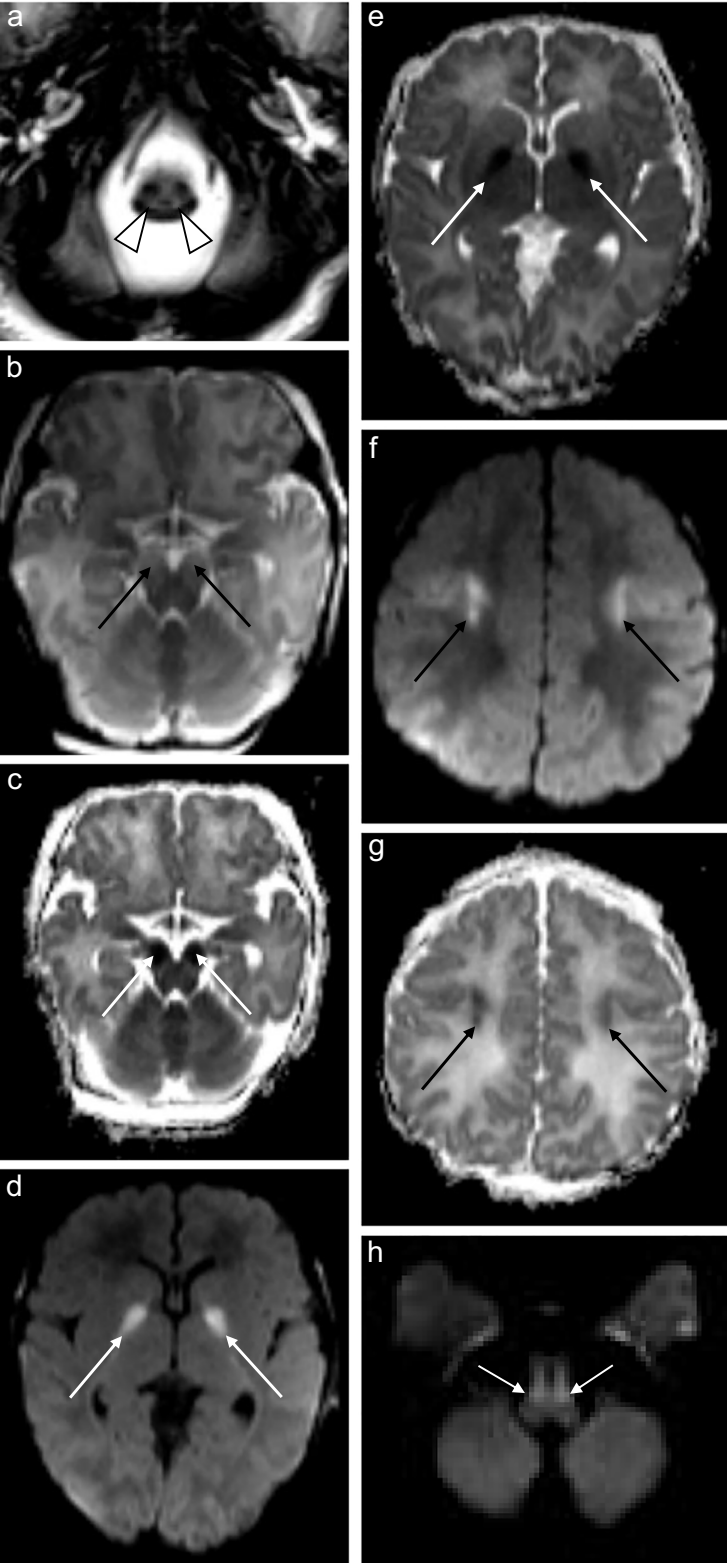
A 2-week-old boy with genetically confirmed *MPV17*-related mitochondrial depletion syndrome. a Axial T2-weighted image shows hyperintense signal in the reticulospinal tracts of the proximal cervical cord (*arrowheads*). b Axial diffusion-weighted image and (c) apparent diffusion coefficient map demonstrate restricted diffusion in the cerebral peduncles (*arrows*). d Axial diffusion-weighted image and (e) apparent diffusion coefficient map demonstrate restricted diffusion in the globus pallidi (*arrows*). f Axial diffusion-weighted image and (g) apparent diffusion coefficient map demonstrate restricted diffusion in the subperirolandic white matter (*arrows*). h Axial diffusion-weighted image shows restricted diffusion in the medullary pyramidal tracts (*arrows*)
